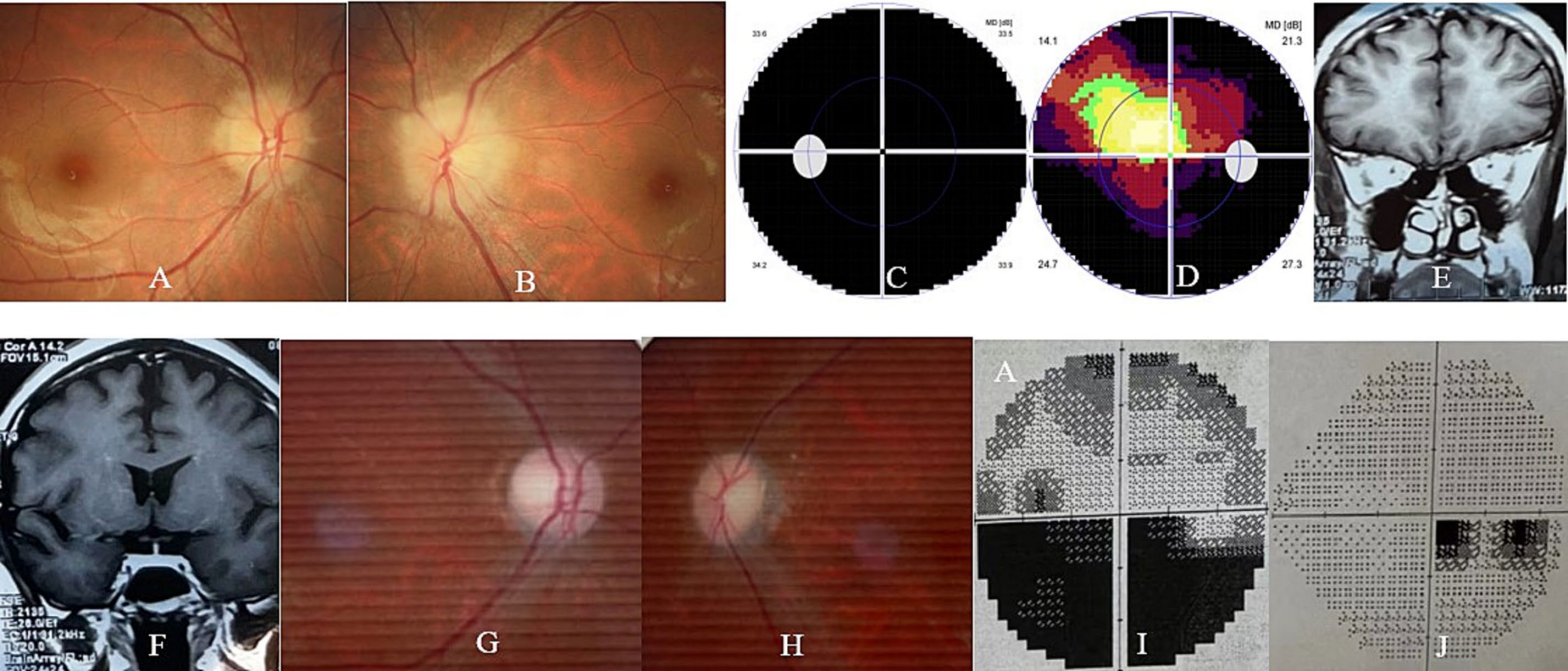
Ophthalmic and orbital MRI examination of the patient. At presentation, the ophthalmic examination revealed bilateral optic disk swelling (A,B) and the visual field test showed diffuse depression (C) and a tubular visual field (D) in the left and right eye, respectively. At the 2-month follow-up, the orbital MRI examination revealed slightly thin bilateral optic nerves (E) and chiasm (F) in both eyes. At the 6-month follow-up, the ophthalmic examination revealed bilateral temporal optic disk pallor (G,H), and the visual field test showed an inferior hemifield defect (I) and a centrocecal scotoma (J) in the left and right eye, respectively.
